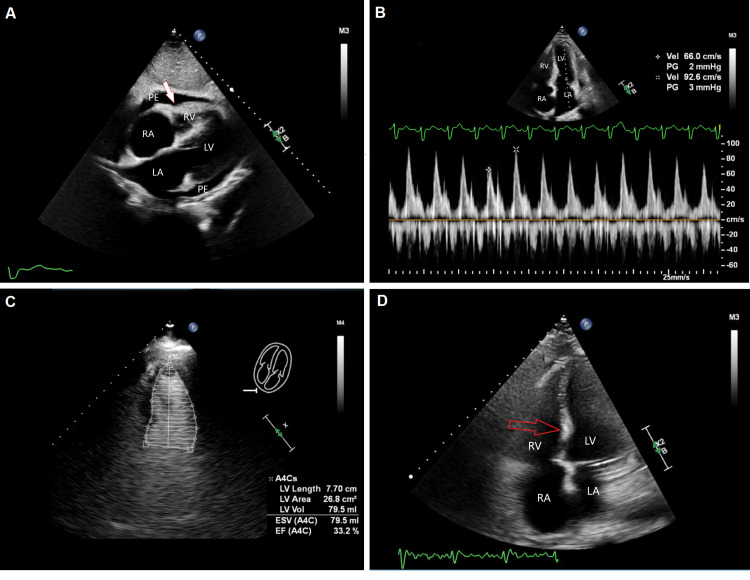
Transthoracic echocardiography A: subxiphoid four-chamber view of the heart demonstrates moderate PE with evidence of early right ventricular end-diastolic compression (marked by white arrow). B: apical four-chamber view pulsed-wave Doppler of mitral valve inflow shows significant variability as an early sign of tamponade physiology. C: after surgical pericardiotomy: contrast echocardiography of left ventricle in the apical four-chamber view shows significantly decreased ejection fraction estimated at 33.2%. D: after surgical pericardiotomy: apical four-chamber view shows (foreshortened) right ventricle with a paradoxical septal shift towards the left (marked by red arrow) RV: right ventricle; LV: left ventricle; RA: right atrium; LA: left atrium; PE: pericardial effusion
